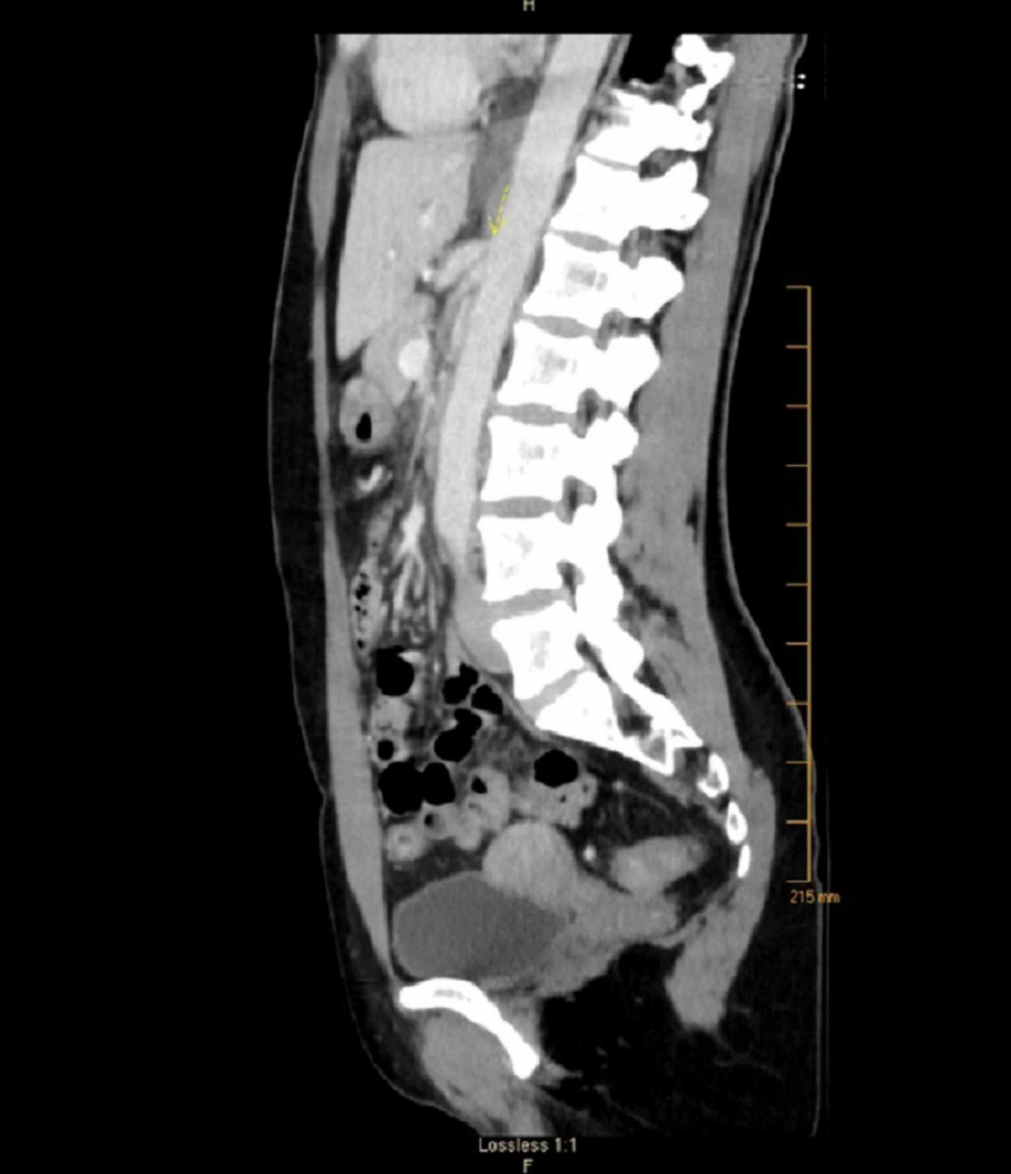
Computed tomography scan, sagittal view, showing severe stenosis of the celiac artery at its take-off from the abdominal aorta (arrow)
